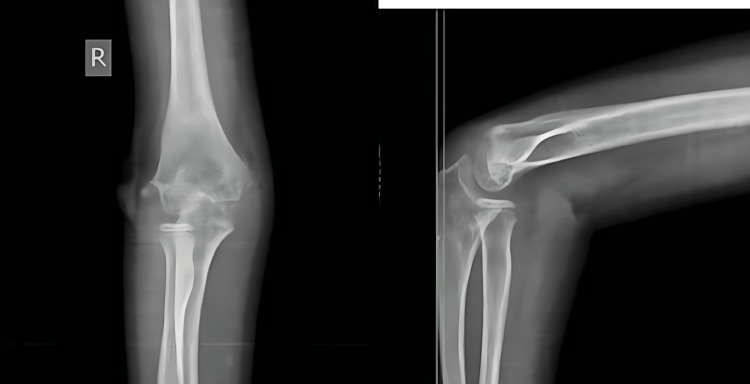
Postoperative X-ray of case 2 after implant removal
